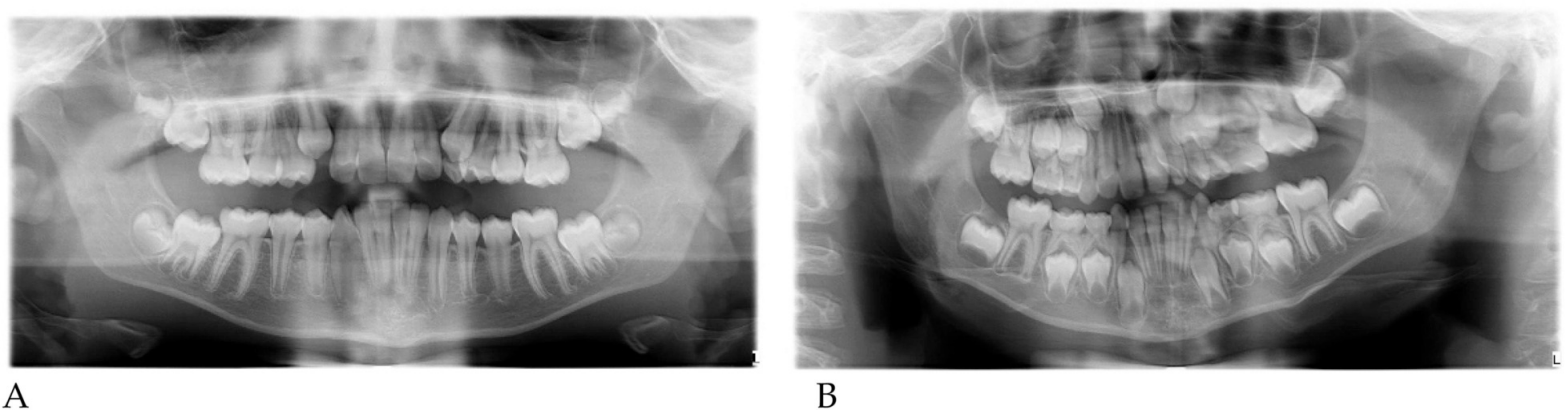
Pre- (A) and post-treatment (B) panoramic radiographs of case 6.
